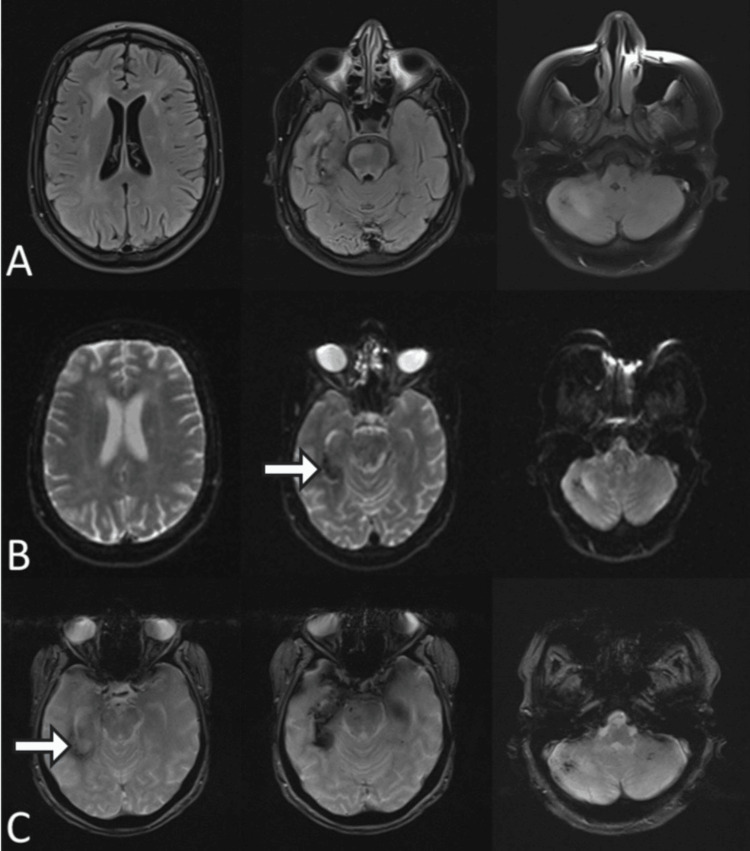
Follow-up brain MRI on day 35 showing near-complete resolution with minimal residual abnormalities (A) Axial FLAIR-weighted images show near-complete resolution of previously described bilateral cortical-subcortical hyperintense lesions, with normalization of signal intensity and resolution of surrounding vasogenic edema. (B) Axial T2-weighted images confirm marked radiological improvement with minimal residual signal abnormalities and no significant mass effect (white arrow). (C) Susceptibility-weighted imaging (SWI)/T2-weighted images demonstrate a marked reduction in previously observed hypointense foci, with minimal residual microhemorrhages (white arrow).
